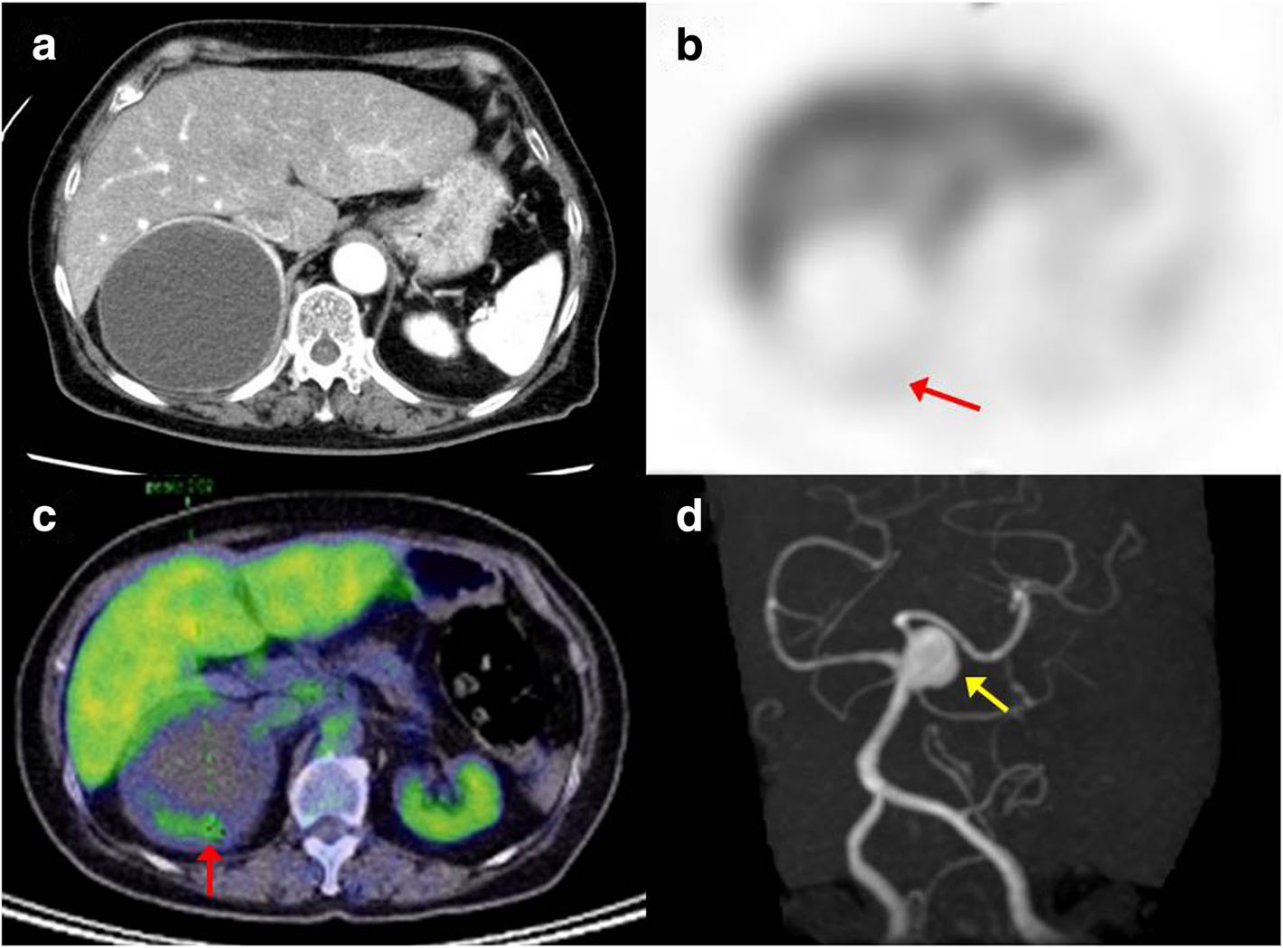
Preoperative findings. a Enhanced computed tomography showed a cystic retroperitoneal tumor with a maximum diameter of 88 mm within the right adrenal gland. It had a walled nodule; a contrast effect was observed in the early phase along the cyst wall, including the nodule. b
